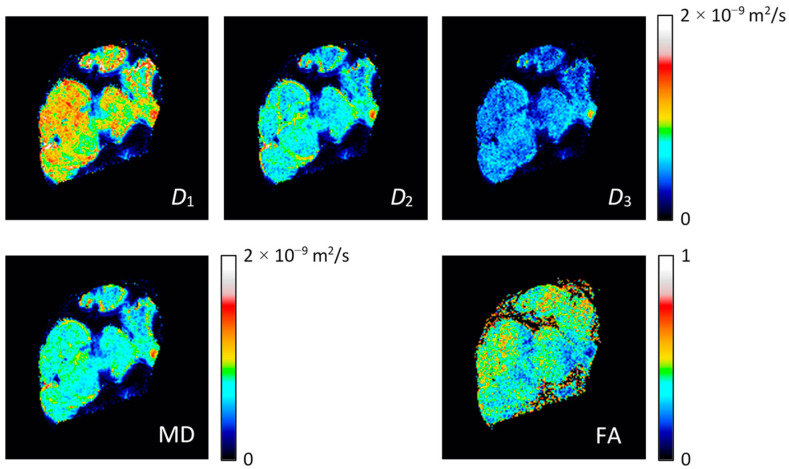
Eigen values of diffusion tensor *D*_1_, *D*_2_ and *D*_3_ (first row) and mean diffusivity MD and fractional anisotropy FA (second row) of the median nerve. The maps were calculated from the DWI data in Figure 2.
